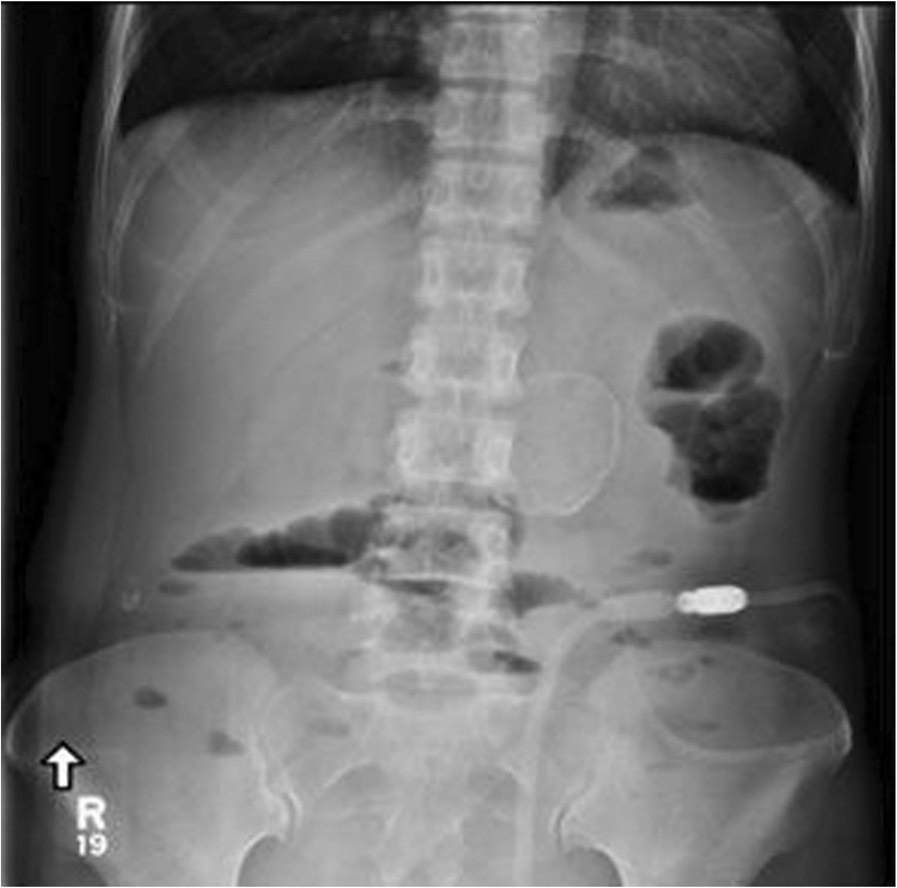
Plain abdominal radiography, upright view, showing several air-fluid levels
